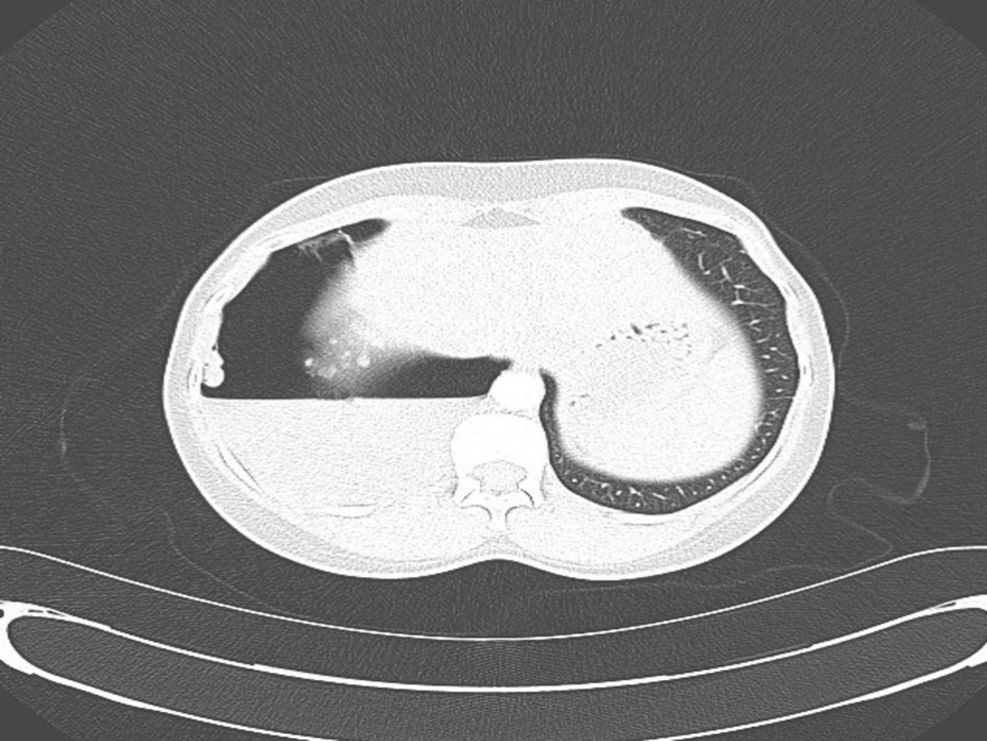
Transverse plane of computed tomography chest showing more clearly unilocular‐dependent fluid with large right hydropneumothorax.
